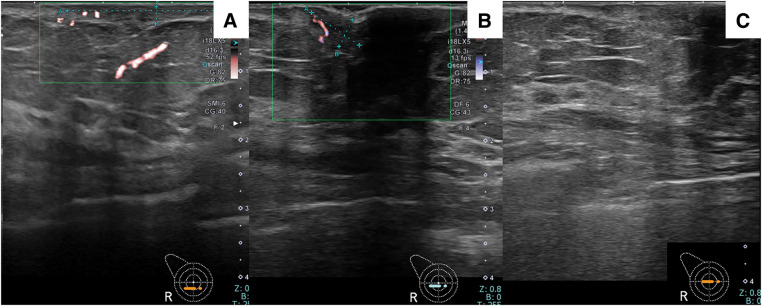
Findings on ultrasonography. (A) Erythematous area showing skin thickening. (B) An 8.4 × 4.3-mm hypoechoic area with blood flow adjacent to the outer side of the nipple. (C) Only scattered 2–3-mm hypoechoic areas were present within the breast. No significant malignant findings were observed.
